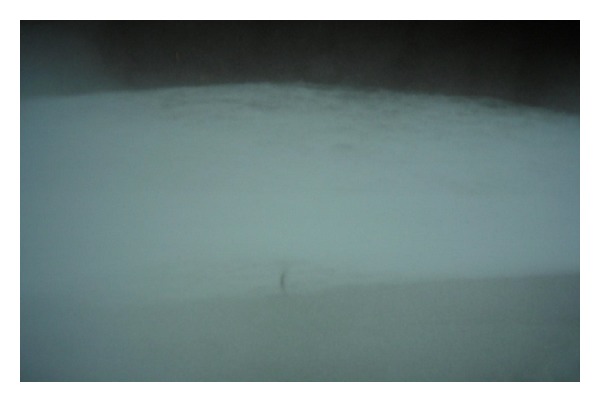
Lateral X-ray of the left tibia-fibula showing cortical lytic defects with no periosteal reaction (‘‘moth-eaten” appearance).
